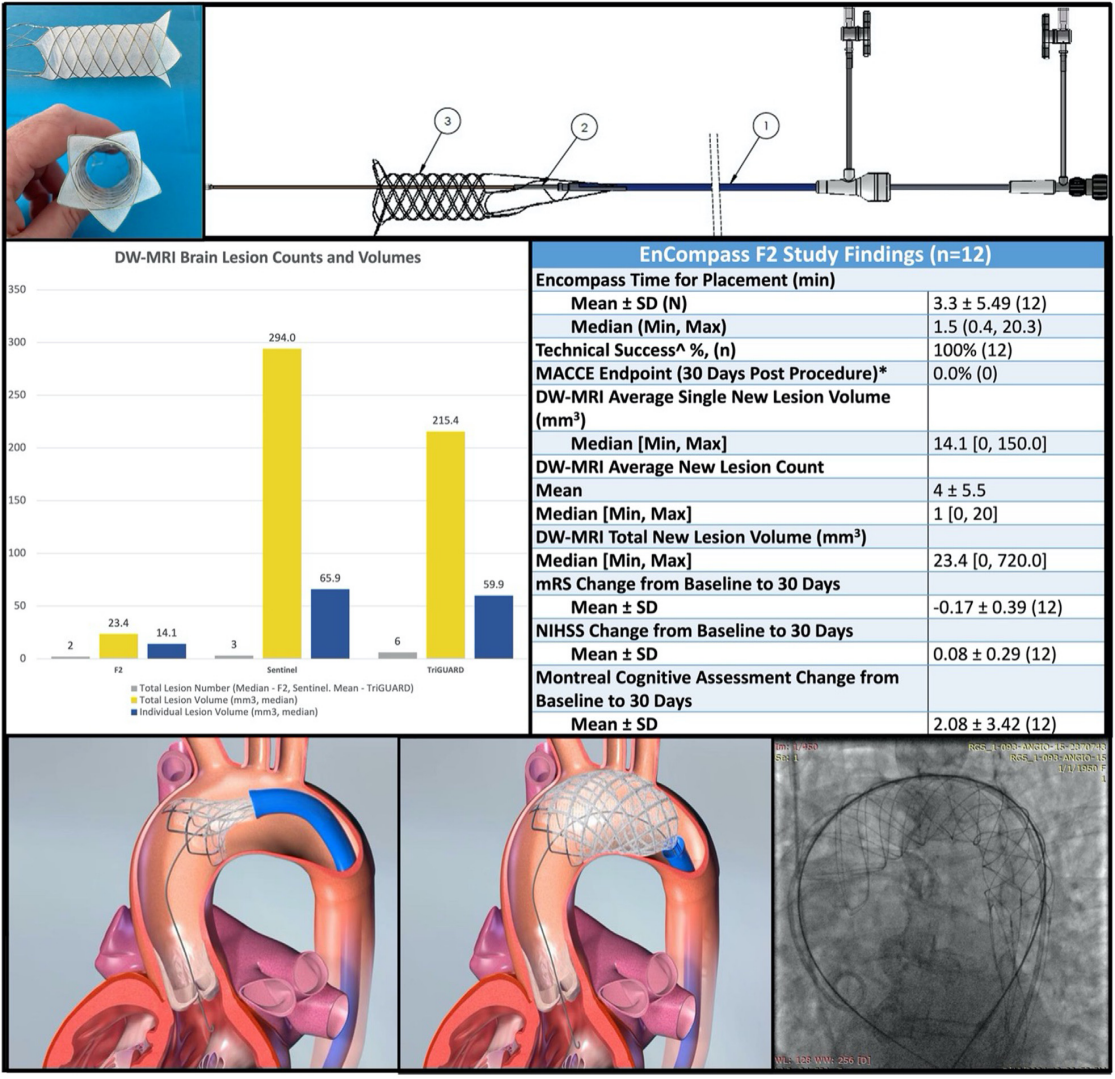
EnCompass F_2_ embolic filter device and FIH study findings. DW-MRI, diffusion-weighted magnetic resonance imaging; MACCE, major adverse cardiac and cerebrovascular event; mRS, modified Rankin scale; NIHSS, National Institutes of Health Stroke Scale.
